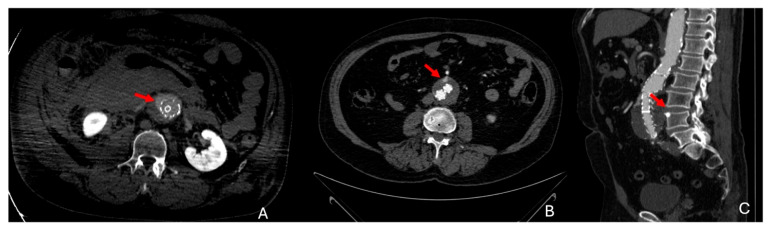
(A) Axial enhanced CTA in arterial phase, showing type 1A endoleak in a patient who had recently undergone EVAR for rAAA (red arrow). (B,C) Axial and sagittal enhanced CTA in arterial phase type 2 endoleak from the IMA (red arrow). EVAR: endovascular aneurysm repair, rAAA: ruptured abdominal aortic aneurysm, IMA: inferior mesenteric artery.
